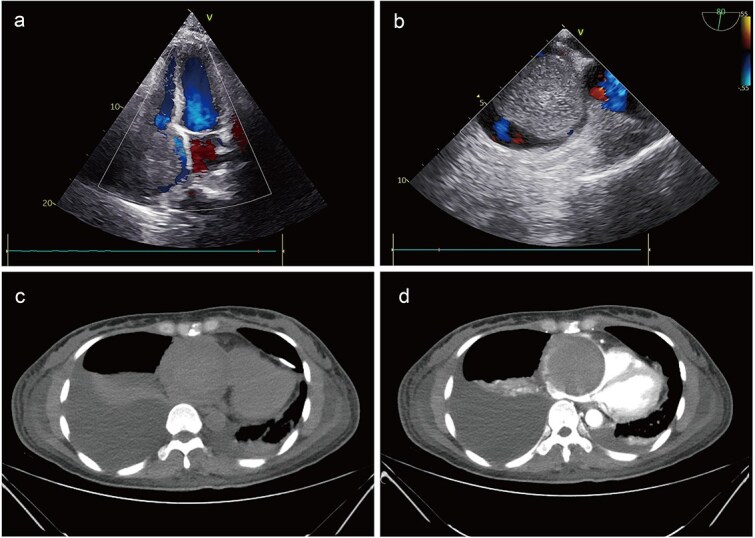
Preoperative imaging. Echocardiography identified a large mass, nearly occupying the entire right atrial cavity (a) and (b). Contrast-enhanced computed tomography showed a right pleural effusion and a right atrial occupying lesion (c) and (d).
